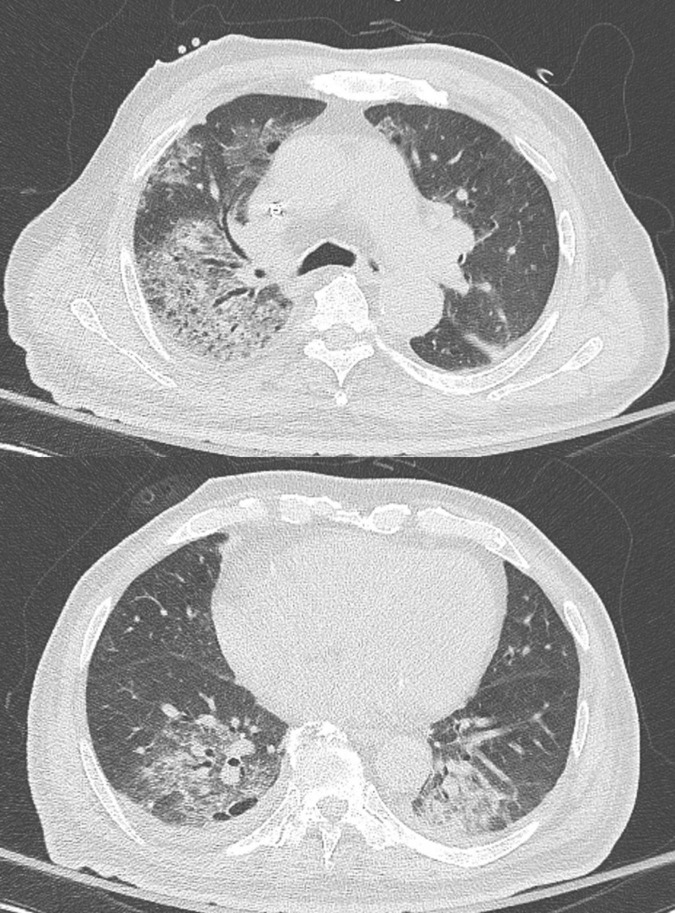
A low dose chest CT shows multifocal peribronchial pneumonic consolidations and ground-glass opacities in right upper lobe and both lower lobes, as well as bilateral pleural effusion.
